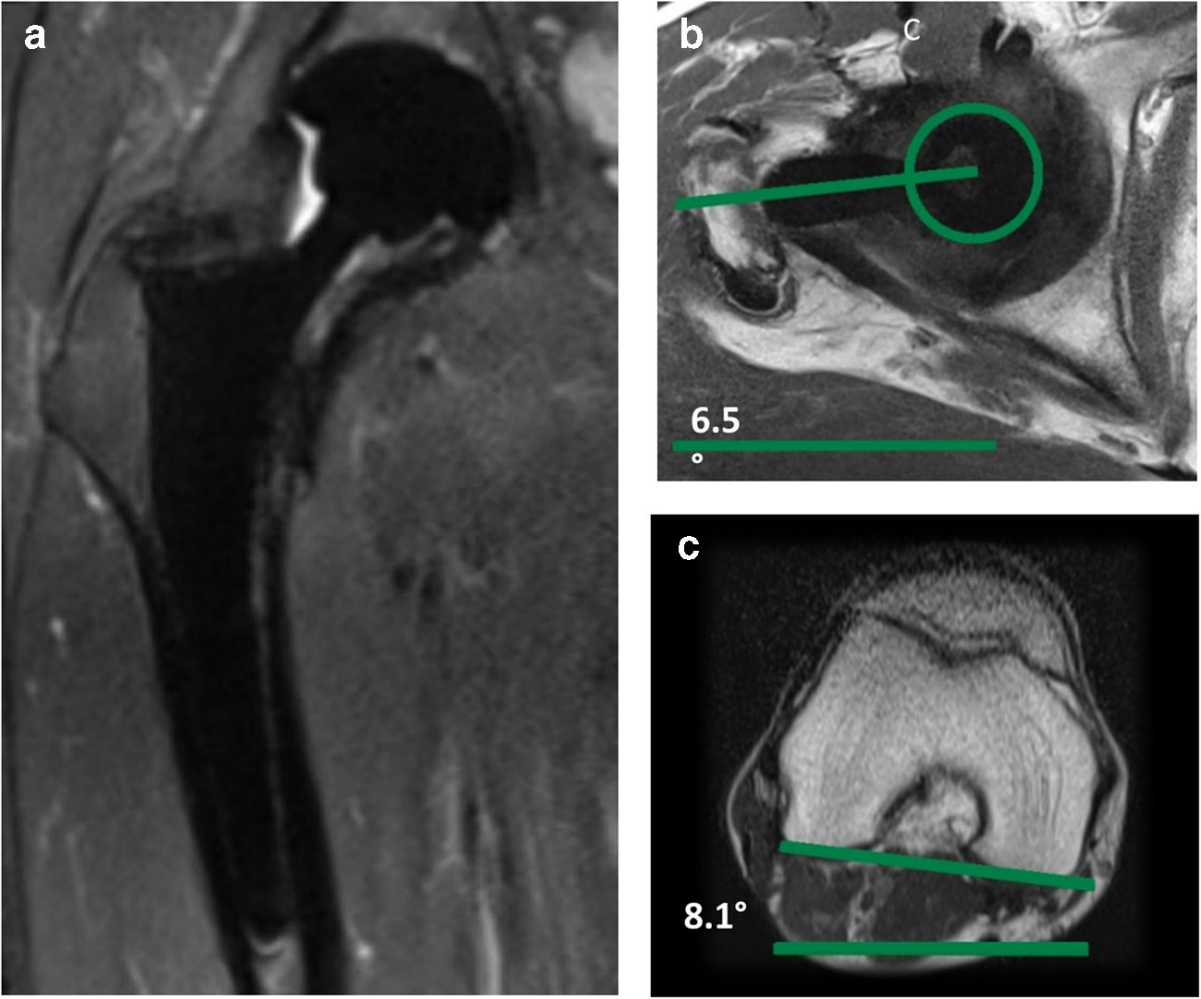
50-year-old male patient with right total hip arthroplasty (THA) on metal artifact suppressed MRI. a Coronal short tau inversion recovery (STIR) image with compressed sensing and slice encoding for metal artifact correction (CS-SEMAC) visualizing the THA with a cementless standard straight stem (S2). b Axial T1-weighted image with high bandwidth at the level of the hip joint with an angle aligned along the neck of the femoral component (green line). c Axial T2-weighted image at the level of the distal femur with a tangent aligned to the posterior femoral condyles (green line). The femoral antetorsion is the combination of the angles in b and c, which was 14.6° in this patient
